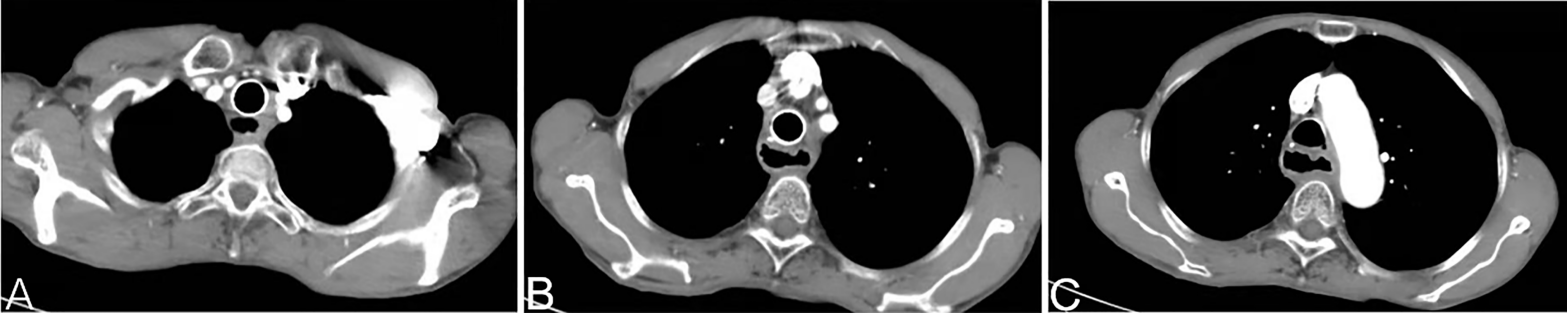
A chest CT at 1 month after arterial infusion chemotherapy shows that the soft-tissue shadow of the esophagogastric anastomosis to be reduced significantly (A–C).
